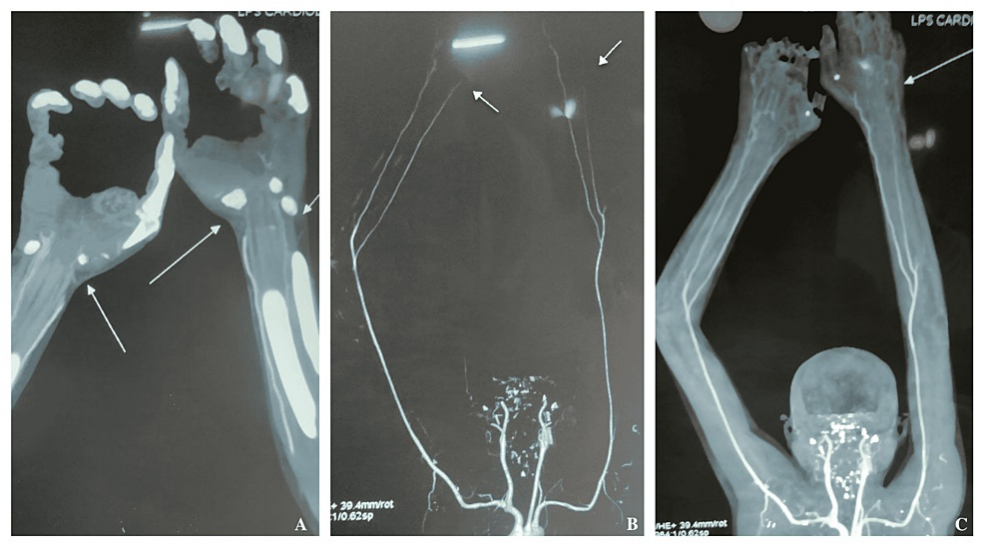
CT angiography showing bilateral poor opacification of palmar and digital arteries (A) and stenosis of left and right distal ulnar artery (B and C).
